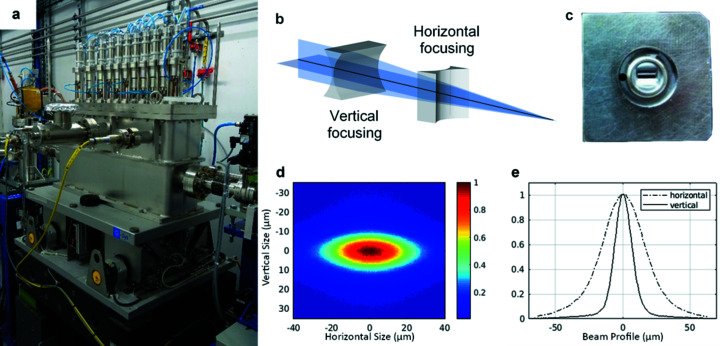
(*a*) The new compact white-beam transfocator vessel. (*b*) Linearly focusing transfocator concept. (*c*) Linear CRL. (*d*) Focal spot size at 50 keV recorded by a high-resolution X-ray imaging camera (pixel size 0.71 µm). (*e*) Measured vertical (solid line) and horizontal (dashed line) beam profiles.
